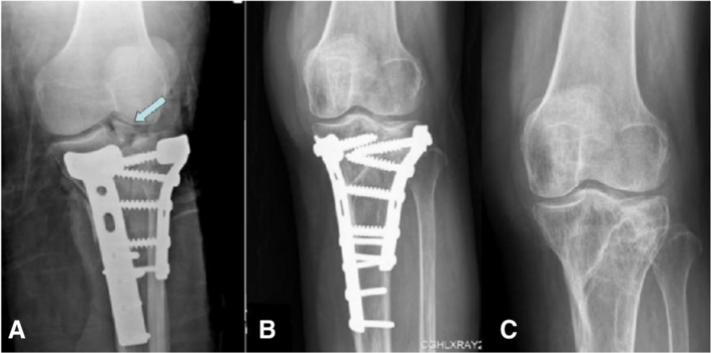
Intraoperative and postoperative plain radiographs (anteroposterior) of the left knee. a A residual tibial eminence avulsion fracture on an intraoperative plain radiograph (*arrow*). b Postoperative plain radiograph showed a good anatomical reduction of tibial plateau and tibial eminence avulsion fractures after fixation. c At the 2-year follow-up, a plain radiograph showed a solid bone union with normal alignment. There were no joint surface depressions or post-traumatic osteoarthritic changes
